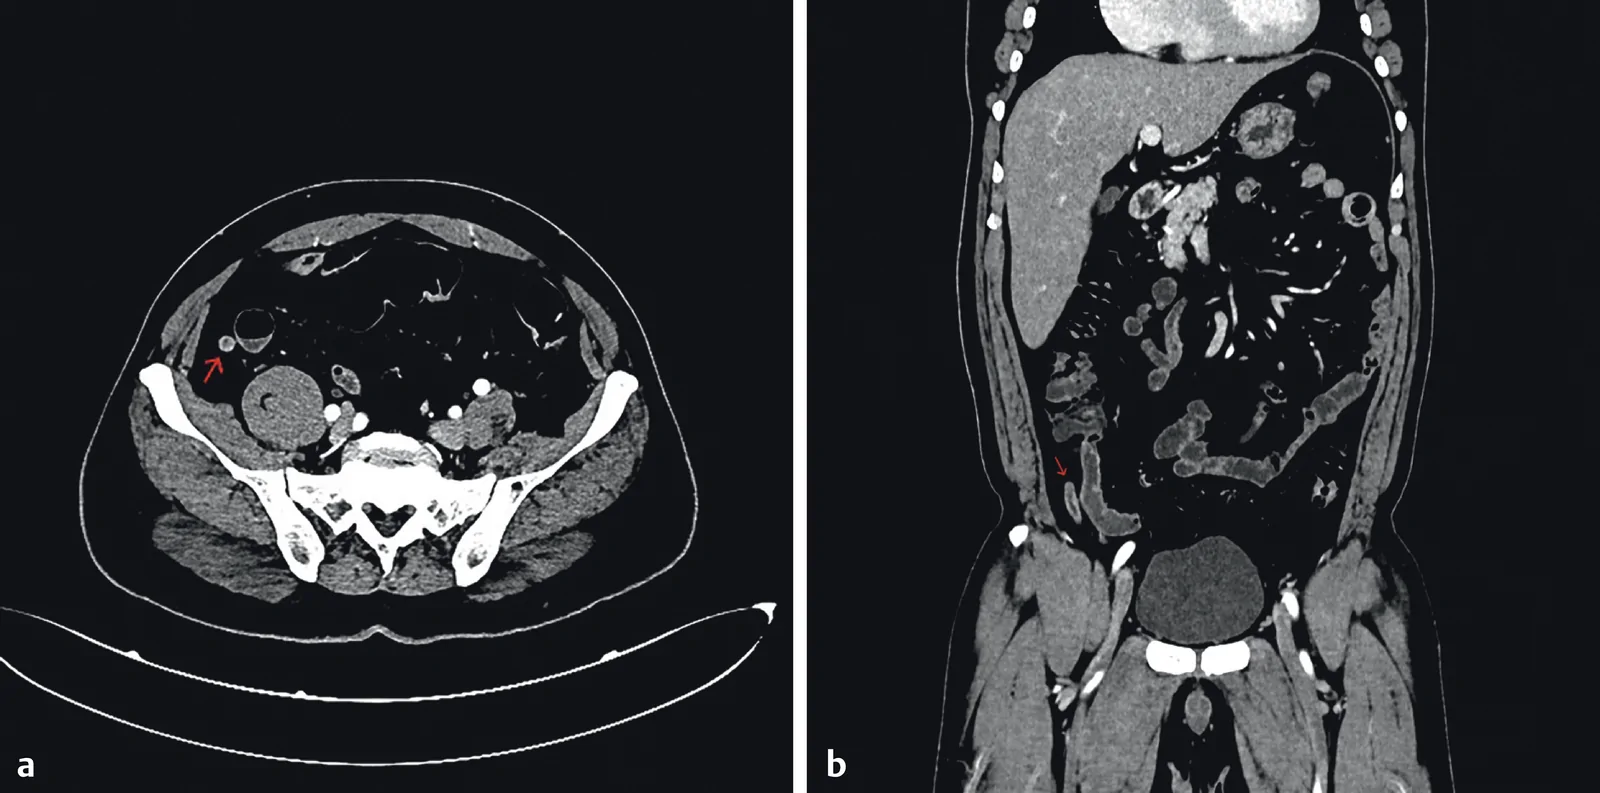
Contrast-enhanced abdominal CT scan. (
